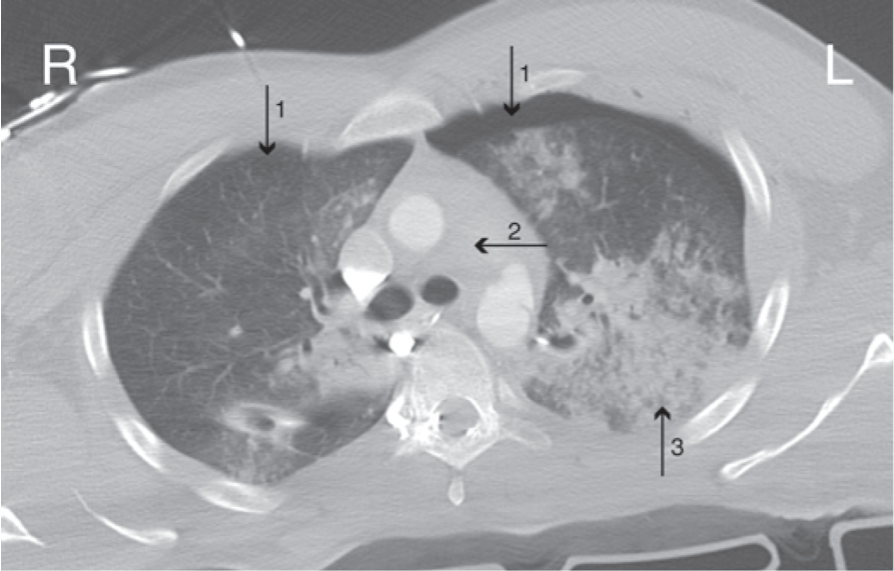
Axial contrast CT chest showed bilateral pneumothoraces (1), periaortic hematoma (2) and pulmonary contusion (3)
